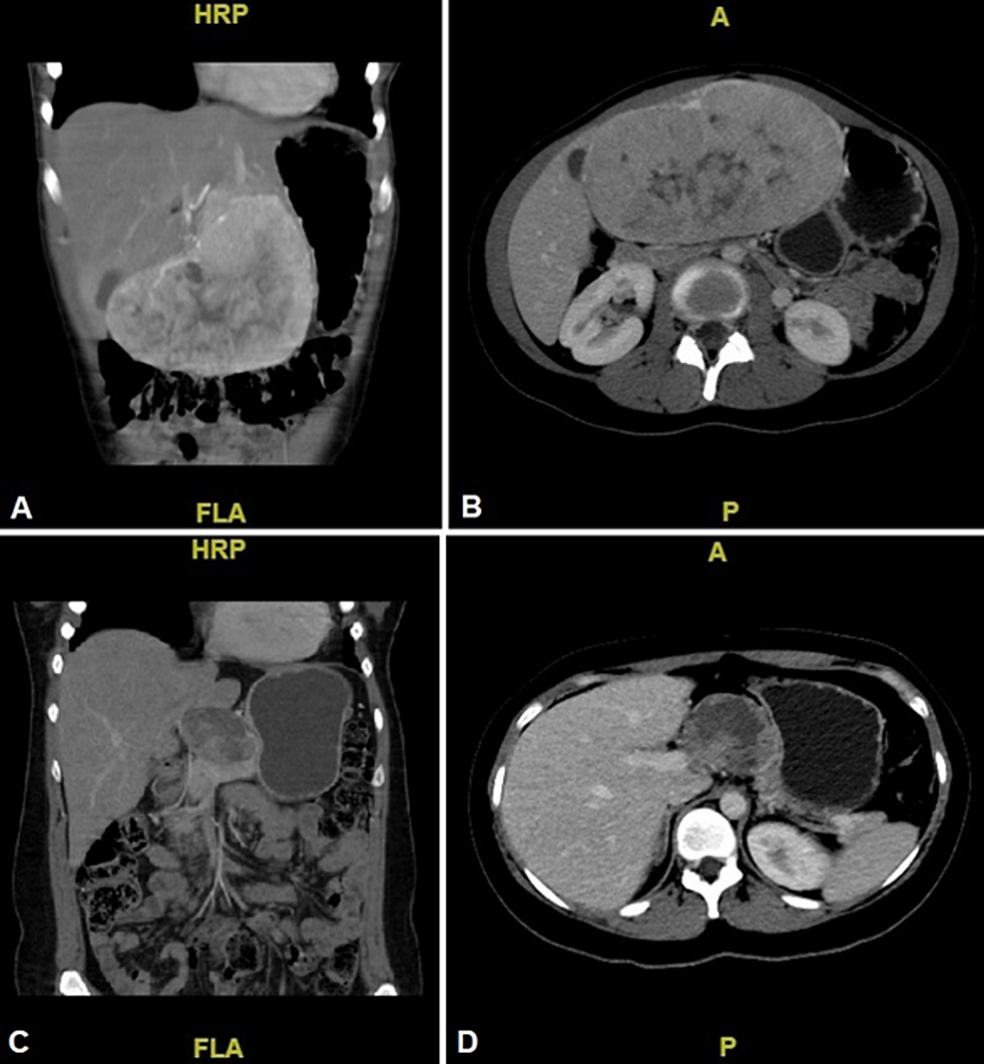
Abdominal CT scan, venous phase A and B: Coronal and axial views showing an extensive solid, heterogeneous, and hypervascular lesion in Segments II and III, with calcifications.
